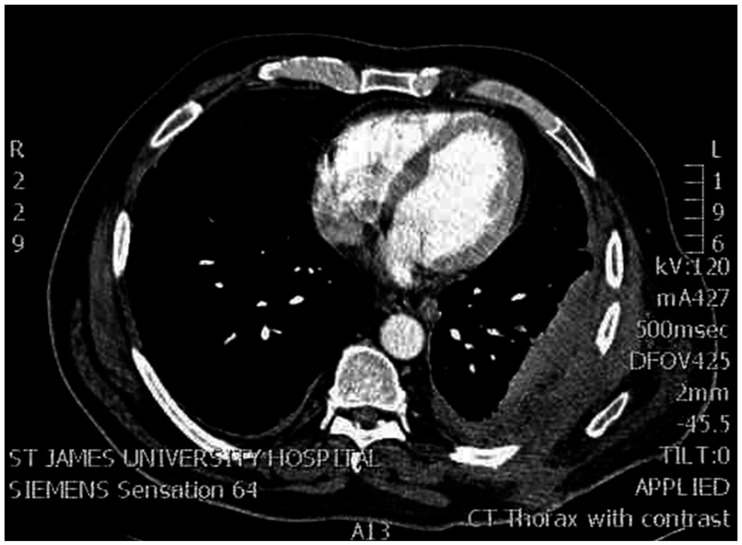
A post-operative CT scan from the archive of our clinic showing complete obliteration of the pleural space and opposition of soft chest wall elements with the partially collapsed left lower lobe.
